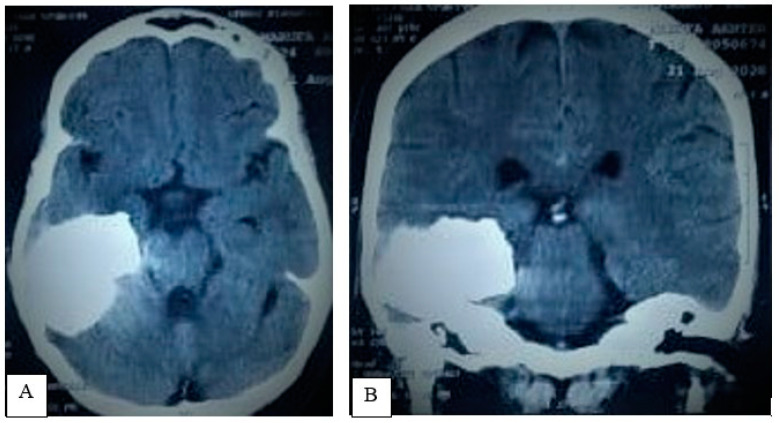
Brain CT scan: axial (A) and coronal (B) images demonstrate an irregular calcified lesion occupying the right middle temporal fossa, anterior to the petrous part of the temporal bone, with minimum mass effect. These features are consistent with middle skull base calcified meningioma.
